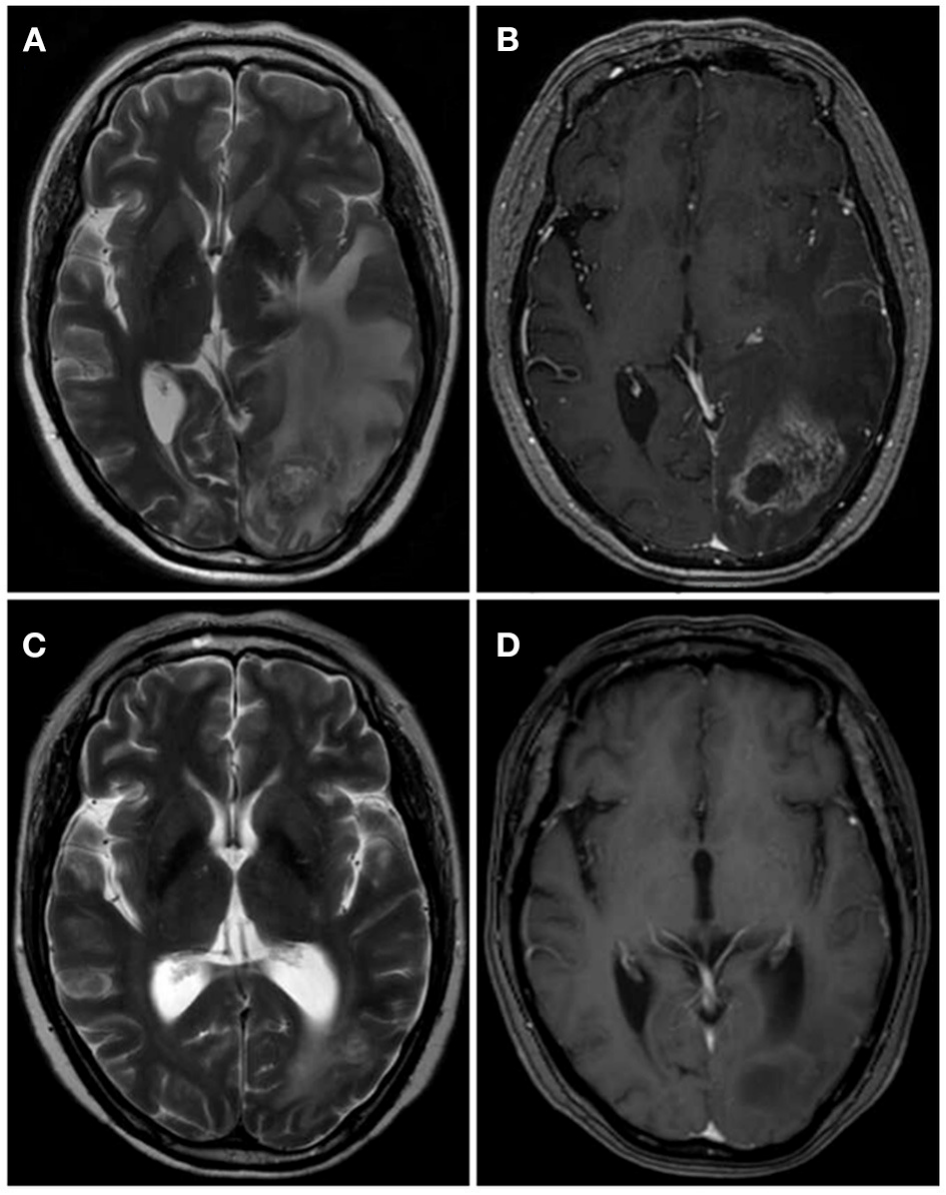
One case of RN after Gamma Knife before and after bevacizumab treatment. (A,B) MRI showed the lesion was located in left occipital with large edema area and obvious enhancement surrounding the tumor. (C,D) After the therapy of bevacizumab (3 mg/kg) for 2 cycles, the zone of lesion reduced dramatically.
